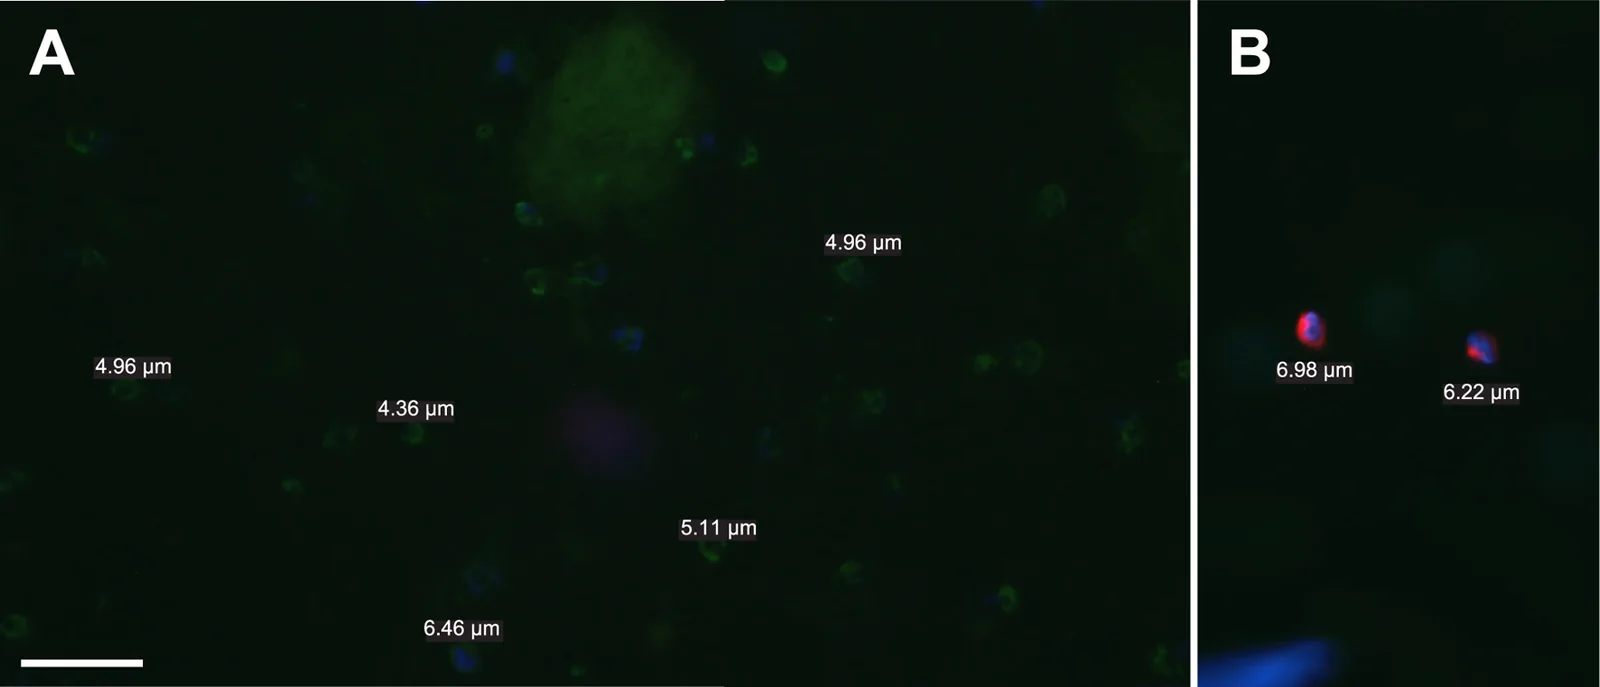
CTCs with diameter smaller than that of a normal prostate epithelial cell appear when the disease progresses to become androgen independent (or mCRPC). Figure legend: PSMA (J591, tumor marker, red), CD45 (leukocyte marker, green), DAPI (nuclear marker, blue). The scale bar equals 20 µm. (A) The diameter of patient leukocytes is between 4.3 µm and 5.2 µm. (B) The diameter of patient CTCs is as small as 6.2 µm-7.0 µm after the disease becomes castrate-resistant.
